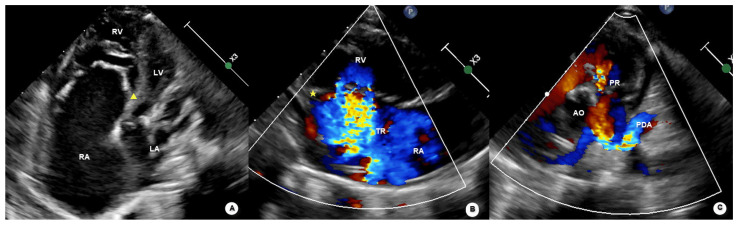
Echocardiographic features of functional pulmonary atresia demonstrating right heart enlargement and ductal-dependent pulmonary blood flow. (A) Apical four-chamber view showing enlargement of the right atrium (RA) and right ventricle (RV), with leftward displacement of the interventricular septum (arrow). (B) Color Doppler imaging demonstrating severe tricuspid regurgitation (TR) associated with right heart dilation. (C) Color Doppler imaging of the pulmonary artery region showing absence of antegrade pulmonary valve flow, with pulmonary blood flow supplied predominantly via a tortuous patent ductus arteriosus (PDA) and minimal pulmonary regurgitation (PR). Abbreviations: RA, right atrium; RV, right ventricle; LA, left atrium; LV, left ventricle; AO, aorta; TR, tricuspid regurgitation; PR, pulmonary regurgitation; PDA, patent ductus arteriosus.
